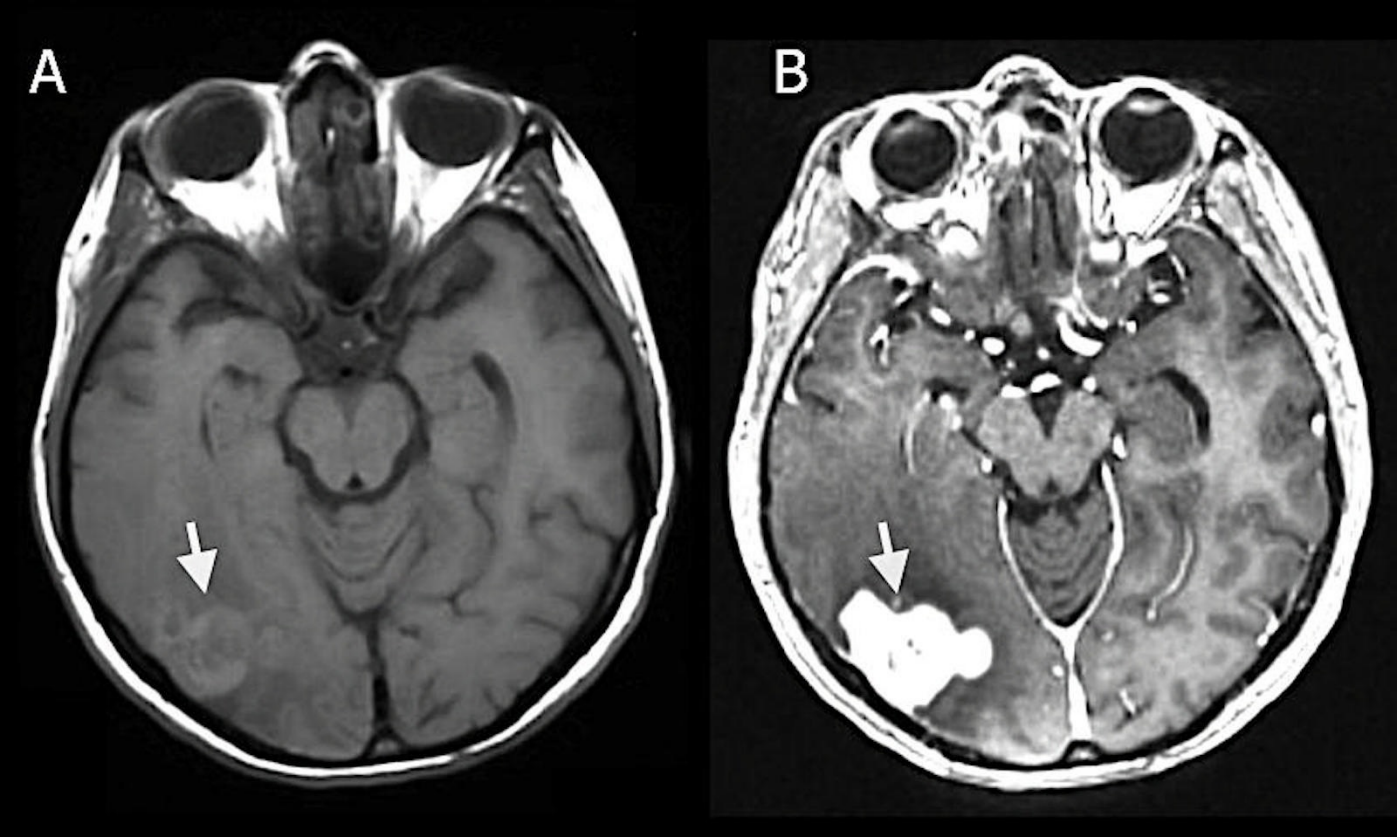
Magnetic resonance imaging T-1 weighted image (MRI T1WI) MRI T1WI Pre (A) and Post (B) gadolinium axial sequences demonstrating a 2 cm x 3 cm, avidly enhancing, lobular, right-parietal mass with leptomeningeal involvement (white arrow).
